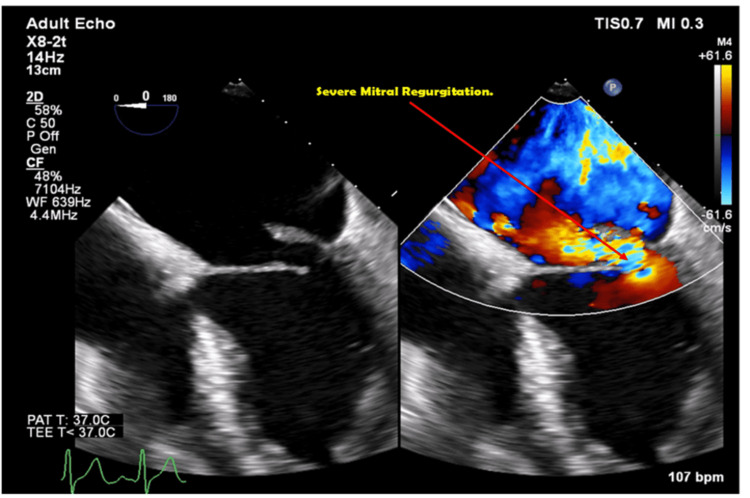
Severe mitral regurgitation on TEE TEE: transthoracic echocardiogram
